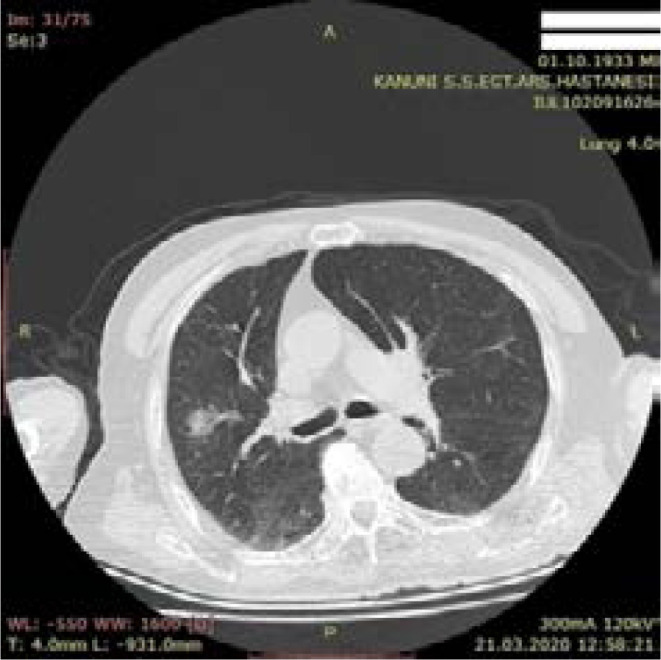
Atypical CT finding - 86-year-old male patient - Dead - Focal ground glass density in right lung upper lobe
